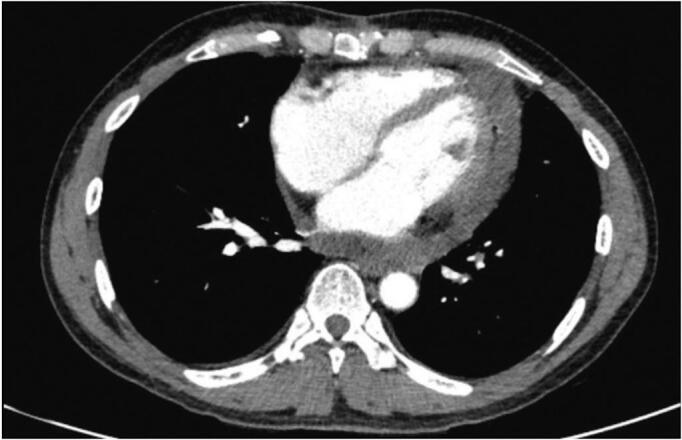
Chest CT (October 2022) with mild pericardial effusion.
